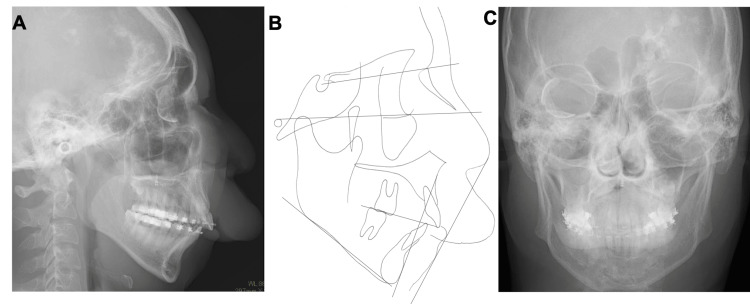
Presurgical evaluation. (A) Lateral cephalometric radiograph. (B) Original tracing of lateral view. (C) Frontal cephalometric radiograph.
